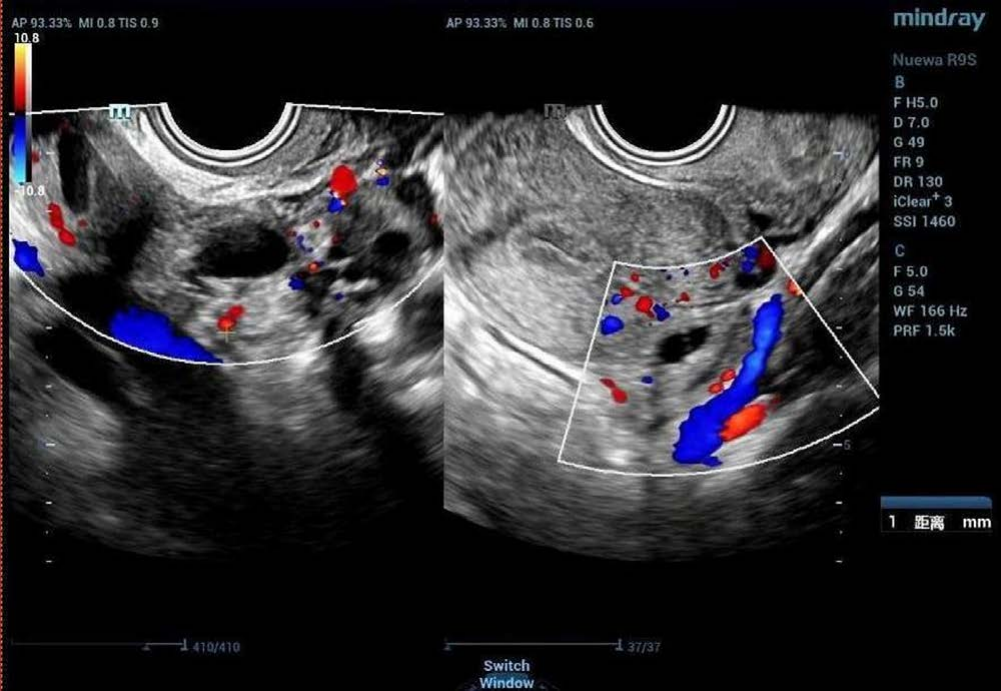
The ultrasound showed a mixed mass in the right adnexa.
